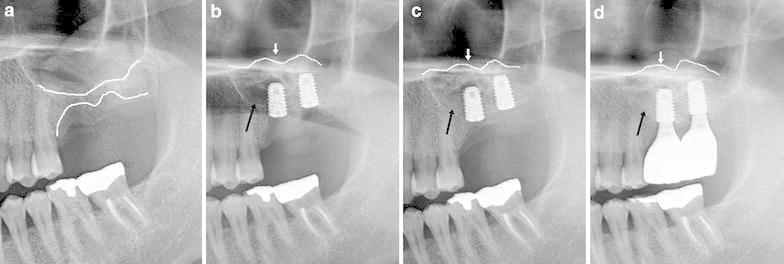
Panoramic radiography of Auto-FDT block with PRP. a Preoperative panoramic view showing severe atrophy in the Lt. maxillary posterior area. b Immediate postoperative panoramic view. Image showing implants placement and Auto-FDT block graft in the sinus area and empty space (radiolucent space) between the Auto-FDT block and residual alveolar bone (*black arrow*). c The graft (after 6 months) showing increased radiopaque in the sinus area (*black arrow*). d After 2 years, there was greater radiopacity in the grafted sinus area using block Auto-FDT and PRP [the final prosthesis without resorption compared with the panoramic view during the uncovering surgery (c, *black arrow*)]. The implants were successful and were fully surrounded by bone. Residual alveolar bone (*white solid line*) and sinus graft height (*white dotted line* and *white arrow*)
